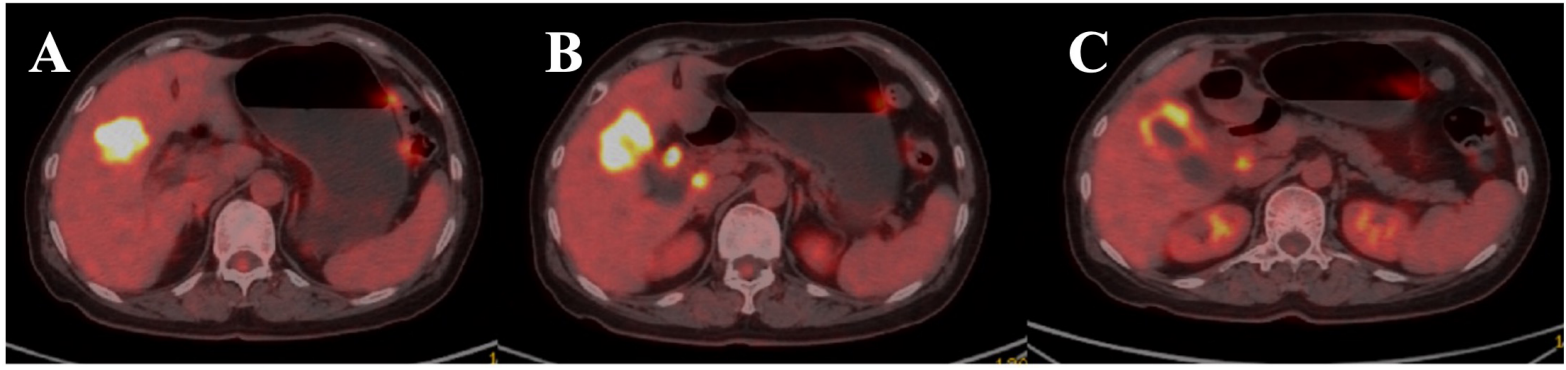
Positron emission tomography-computed tomography (PET-CT) axial image of the upper abdomen. (A) Hypermetabolic lesions in the gallbladder (GB), intrahepatic regions. (B, C) hepatoportal lymph nodes (HP LNs) are shown in the image.
